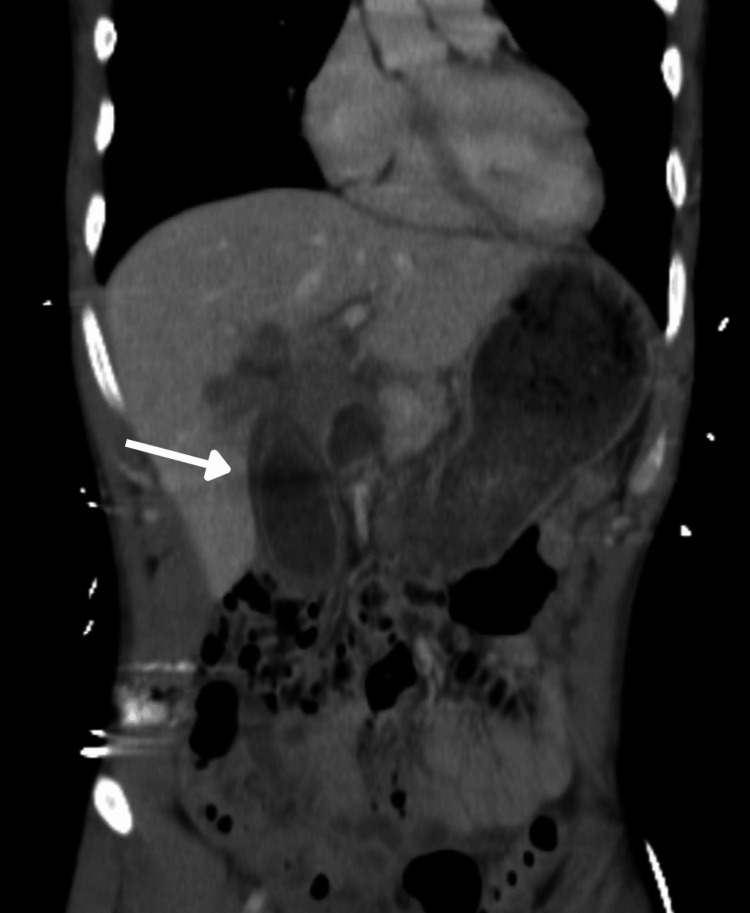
Coronal computed tomography showing wall thickening and mild pericholecystic stranding (arrow)
